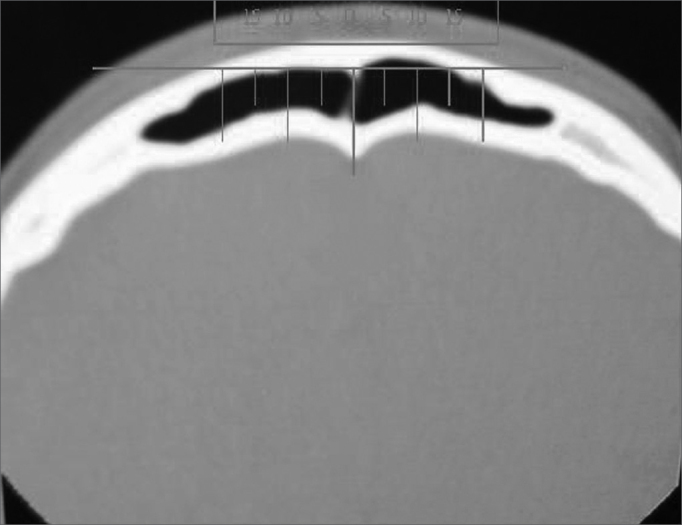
CT-scan axial view with measurements at 5, 10 and 15mm in relation to the midline (crista galli).
